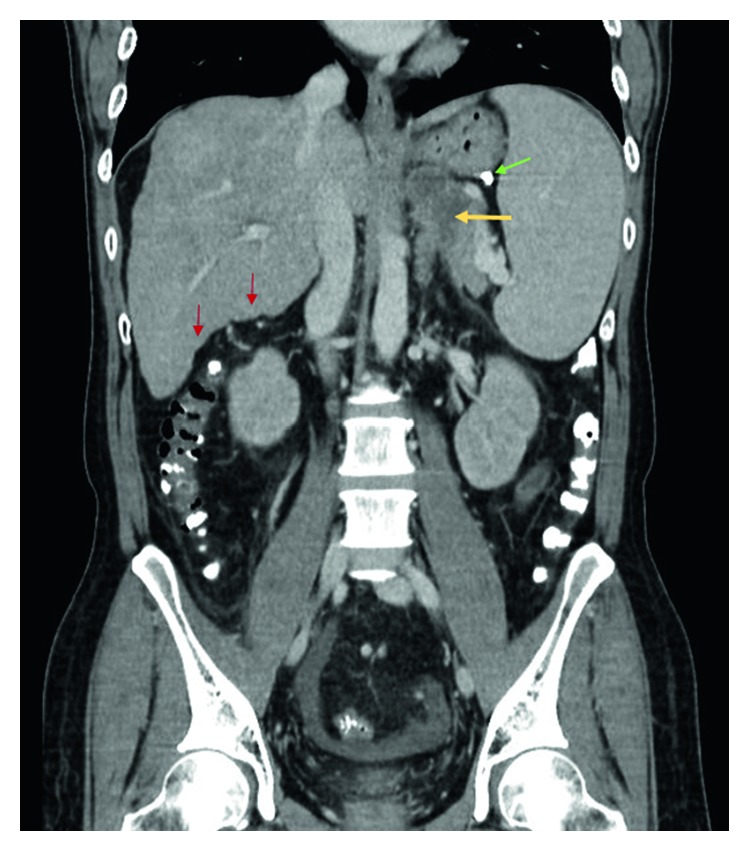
Computed tomography view of the abdomen (in coronal view). The liver contour is nodular (red arrows) confirming the presence of cirrhosis. There is a hypodense collection (yellow arrow) near the splenic hilum and below the stomach, corresponding to the retrogastric abscess. The small amount of residual N-butyl-2-cyanoacrylate is brightly visible in the perigastric region (green arrow).
